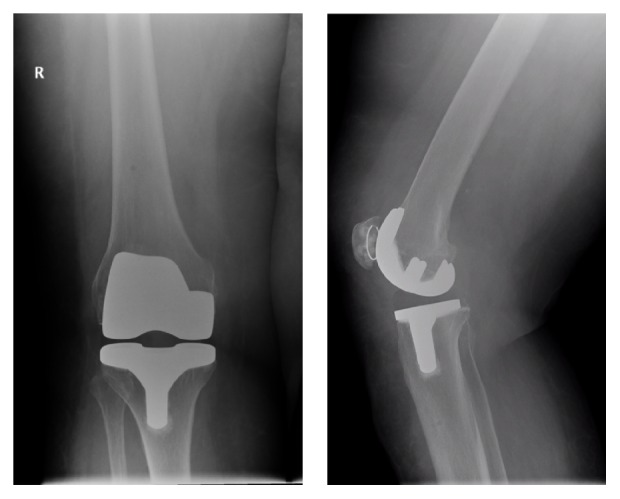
Postoperative AP and lateral radiographs of right Columbus TKR.
